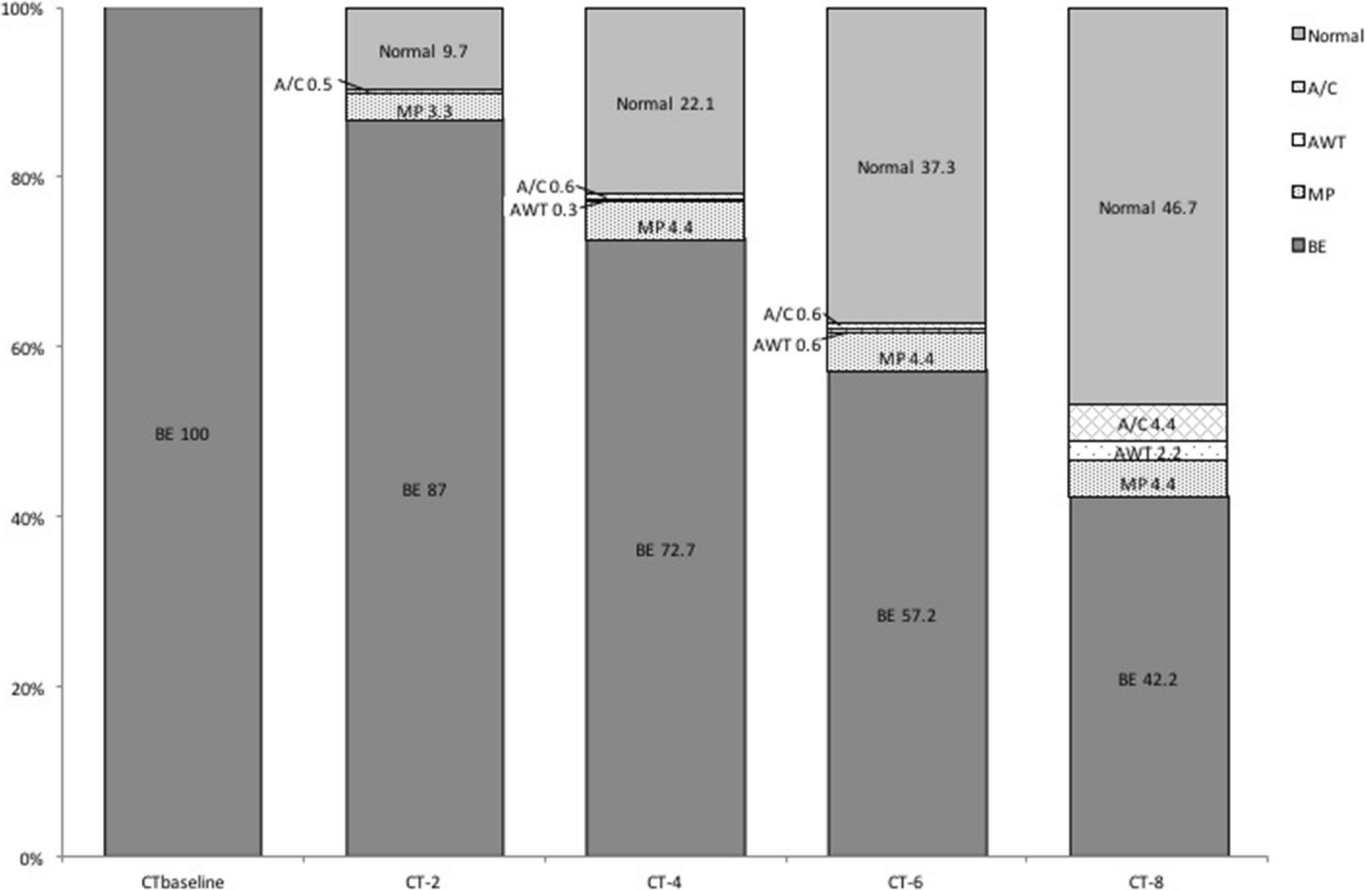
Structural lung damage over time (n = 362 in 43 patients). Dark grey represents bronchiectasis, light-dark grey (area above dark grey) represents mucus plugging, grey dots represent airway wall thickening, white dots represent atelectasis/consolidation, light grey (on top) represents normal airways. CT_baseline_ is the most recent CT
